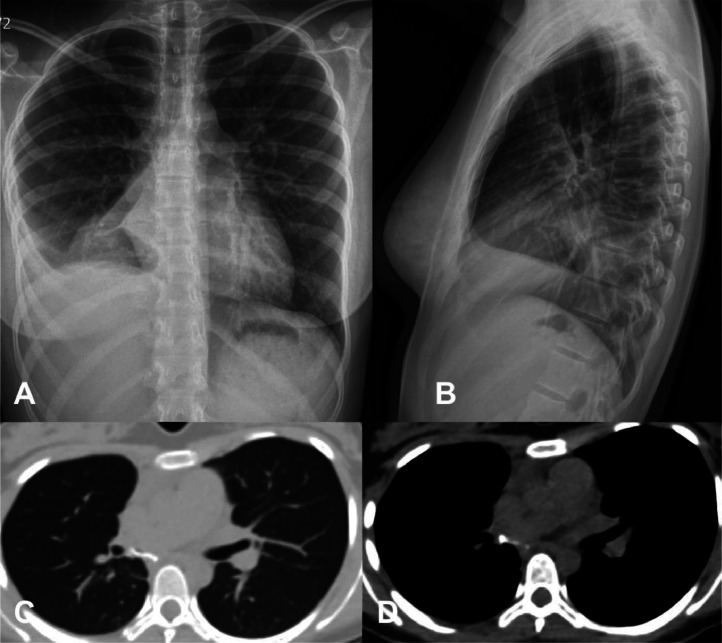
Posteroanterior (PA) and lateral thoracic radiographs (A-B) demonstrate postsurgical changes following a right lobectomy, with obliteration of the right costophrenic angle due to a larger volume of effusion on the right side and partial atelectasis of the middle lobe. Axial chest CT images in lung windows without contrast (C-D) confirm postsurgical changes, including volume loss and a rightward mediastinal shift. A triangular-shaped paracardiac opacity is consistent with partial middle lobe atelectasis. Additionally, obliteration of the right costophrenic angle is observed, likely secondary to residual pleural effusion.
